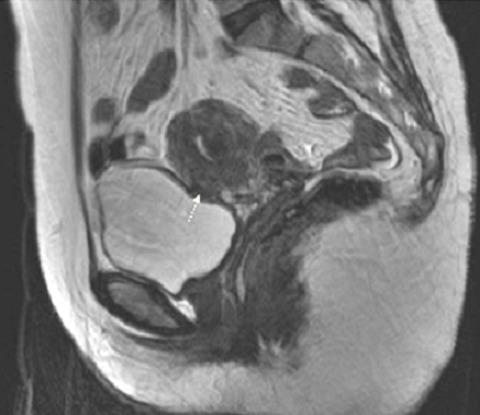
Pelvic MRI: sagittal sequence (T2 FSE) evidenced an oblong nodular lesion in hyposignal T2 in front of the vesicouterine cul de sac
